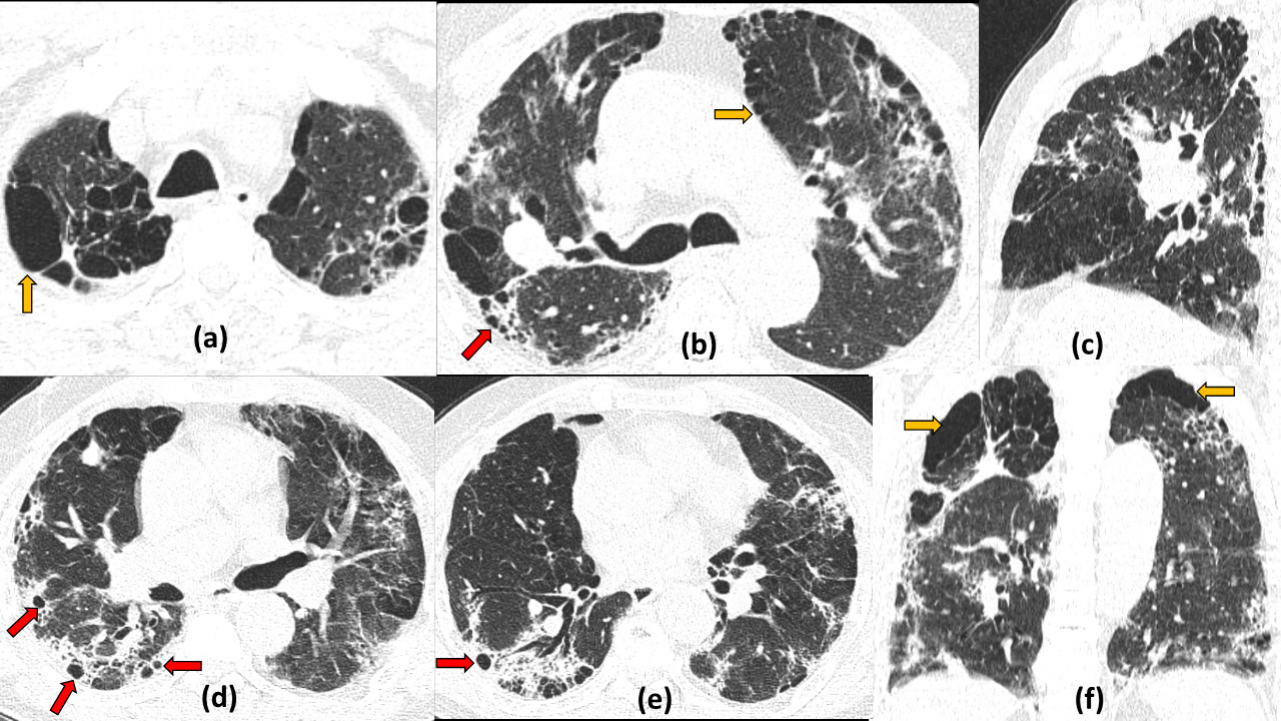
Emphysematous bullae with cystic air spaces as COVID19 sequelae: 67-year-old smoker and a known case of COPD with COVID19 illness. Axial (a, b, d, e), sagittal (c) and coronal (f) CT sections on Day 20 of illness, show multiple tiny relatively thick-walled cystic air spaces (red arrows) noted both in the subpleural and intralesional location, seen along the distribution of the post-COVID19 fibrotic changes in the bilateral peripheral lung fields. In addition, larger thin-walled emphysematous bullae/blebs are seen in the upper sections of the chest (yellow arrows) largely confined to areas uninvolved with the fibrotic changes. These bullae are possibly due to pre-existing COPD (However, no previous HRCT was available for comparison). COPD, chronic obstructive pulmonary disease.
